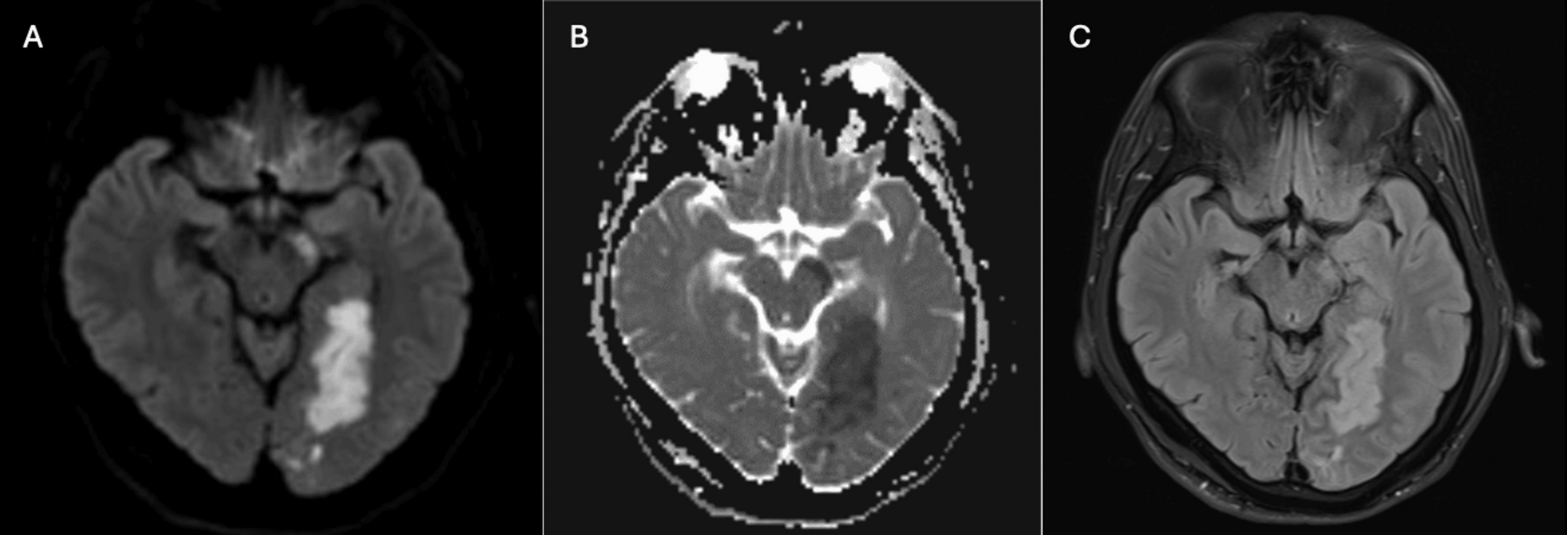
MRI showing areas of brain infarcts Acute left posterior cerebral artery territory infarcts are seen involving the left occipital lobe, left temporal region along para-hippocampal gyrus, left crus of midbrain, and left red nucleus. This is seen in (A) diffusion-weighted imaging, (B) apparent diffusion coefficient,  and (C) fluid-attenuated inversion recovery images.
